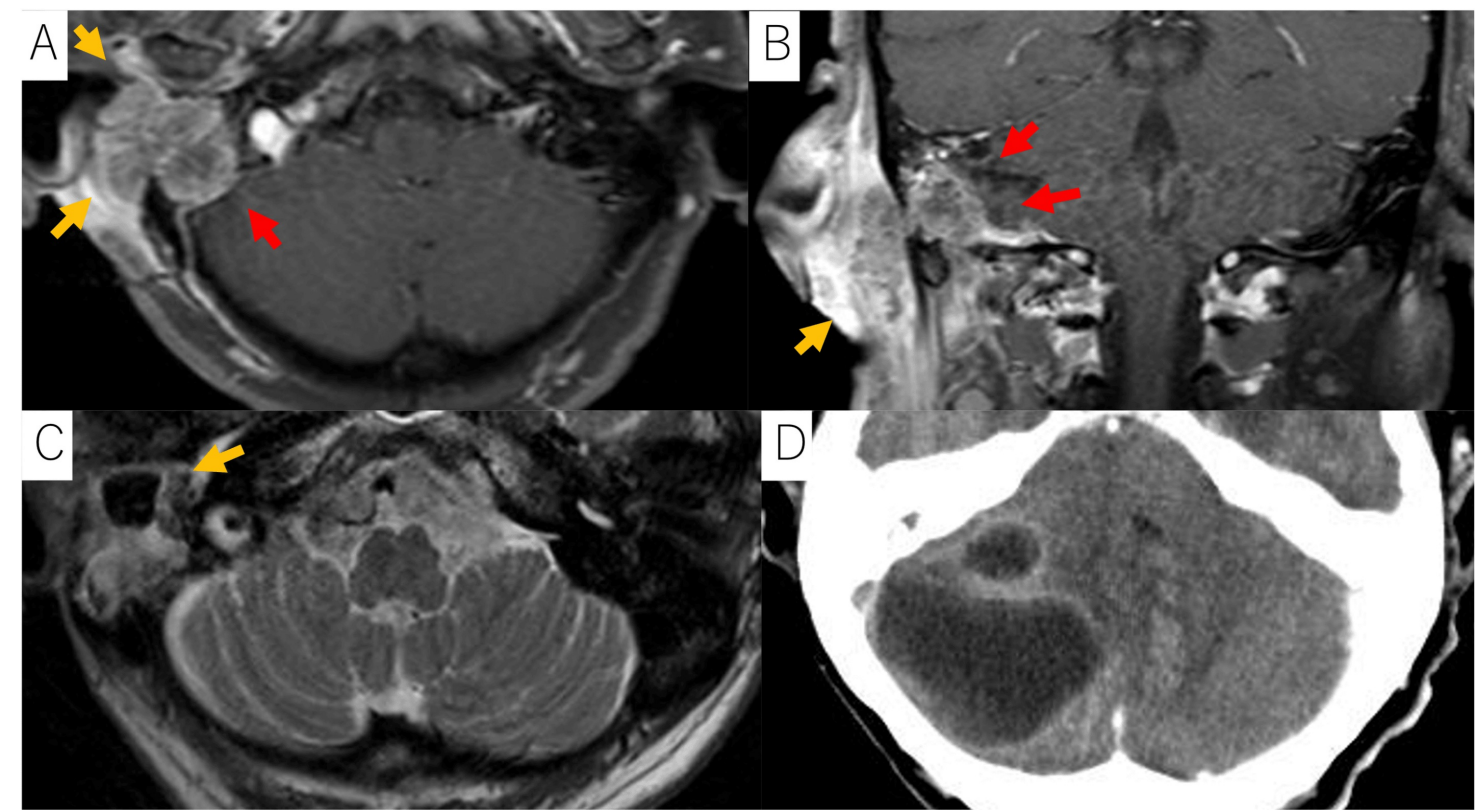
Images of the brain abscess in case 1 A, B: Magnetic resonance imaging (MRI) T1-weighted with gadolinium enhancement images demonstrating the tumor (indicated by yellow arrows and red arrows). Additionally, the image demonstrates tumor invasion into the right sigmoid sinus and dura mater (indicated by red arrows). The tumor was classified as T4 based on the modified Pittsburg classification. There was no cervical lymph node involvement or distant metastasis. C: MRI T2-weighted image reveals destruction of the right temporal bone, observed seven years post-radiation therapy (RT) (indicated by yellow arrow). D: Computed tomography (CT) scan demonstrates a ring-enhancing lesion with a low-density area in the right cerebellum, consistent with a cerebellar abscess that developed eight years post-radiotherapy. The abscess measured a maximum of 43 mm. It was managed with drainage and antibiotic therapy. The patient exhibited no evidence of recurrence or subsequent brain abscess formation.
